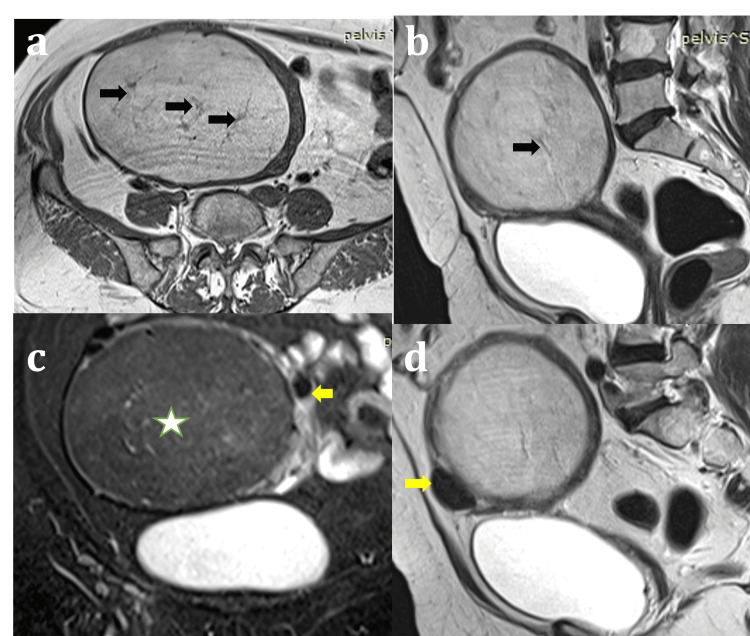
MRI features of uterine lipoleiomyoma Axial T1-weighted (a), sagittal T2-weighted (b), and coronal STIR (c) MR images demonstrating a homogenously T1- and T2-hyperintense lesion arising from the uterine fundus, containing a few internal soft tissue strands (black arrows) and showing complete suppression on fat-saturated sequence (asterisk). Additional T2-hypointense intramural nodules are noted along the periphery of the lesion (yellow arrows) (c, d). STIR: short tau inversion recovery, MRI: magnetic resonance imaging
